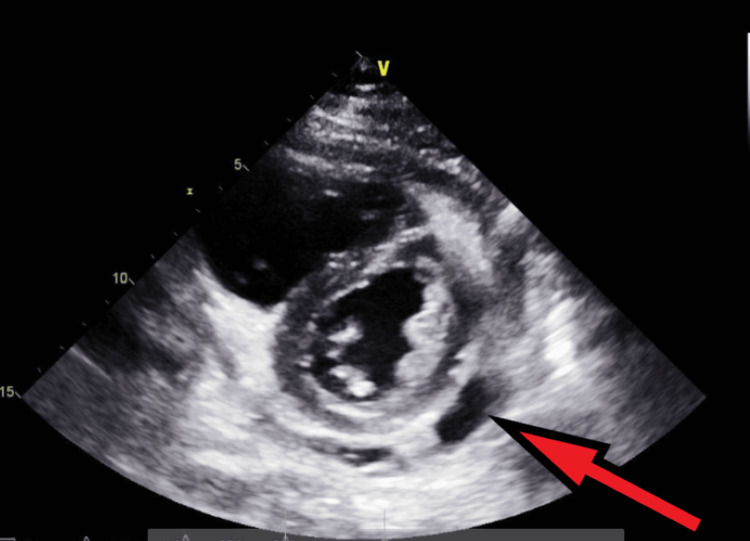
Transthoracic echocardiography post-pericardiocentesis - image 2 Transthoracic echocardiography post-pericardiocentesis; parasternal short axis view shows a decreased amount of pericardial fluid collection (red arrow) with the presence of some loculations
